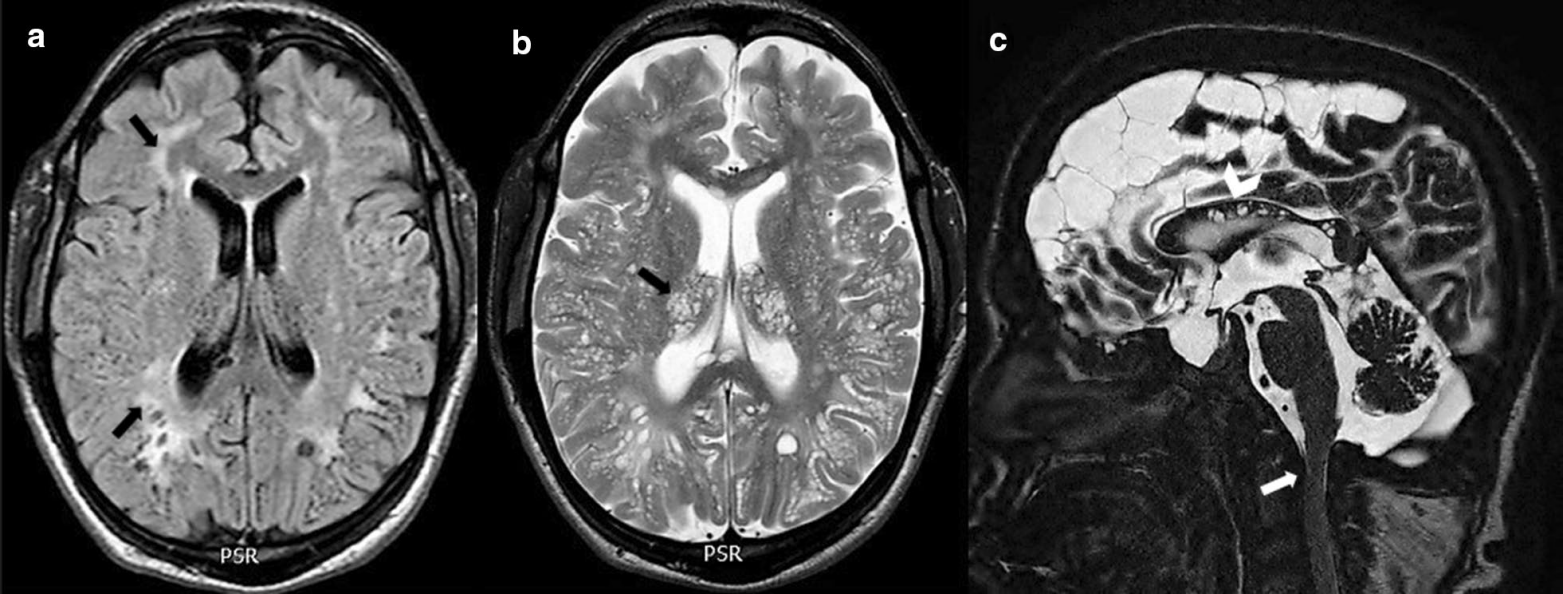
Brain MRI scans of a 20-year-old male patient affected by MPS II (attenuated form). Axial FLAIR image a shows periventricular and subcortical (arrows) white matter lesions (lesion load = 1.8%). Axial T2-weighted image b shows dilated perivascular spaces (score 3) prominently seen in the thalami and basal ganglia (arrow) and enlargement of subarachnoid spaces. Midsagittal T2-weighted image c shows dilated perivascular spaces within the corpus callosum (arrowhead), and effacement of CSF (arrow) and spinal stenosis at C1–C2 (score 1)
